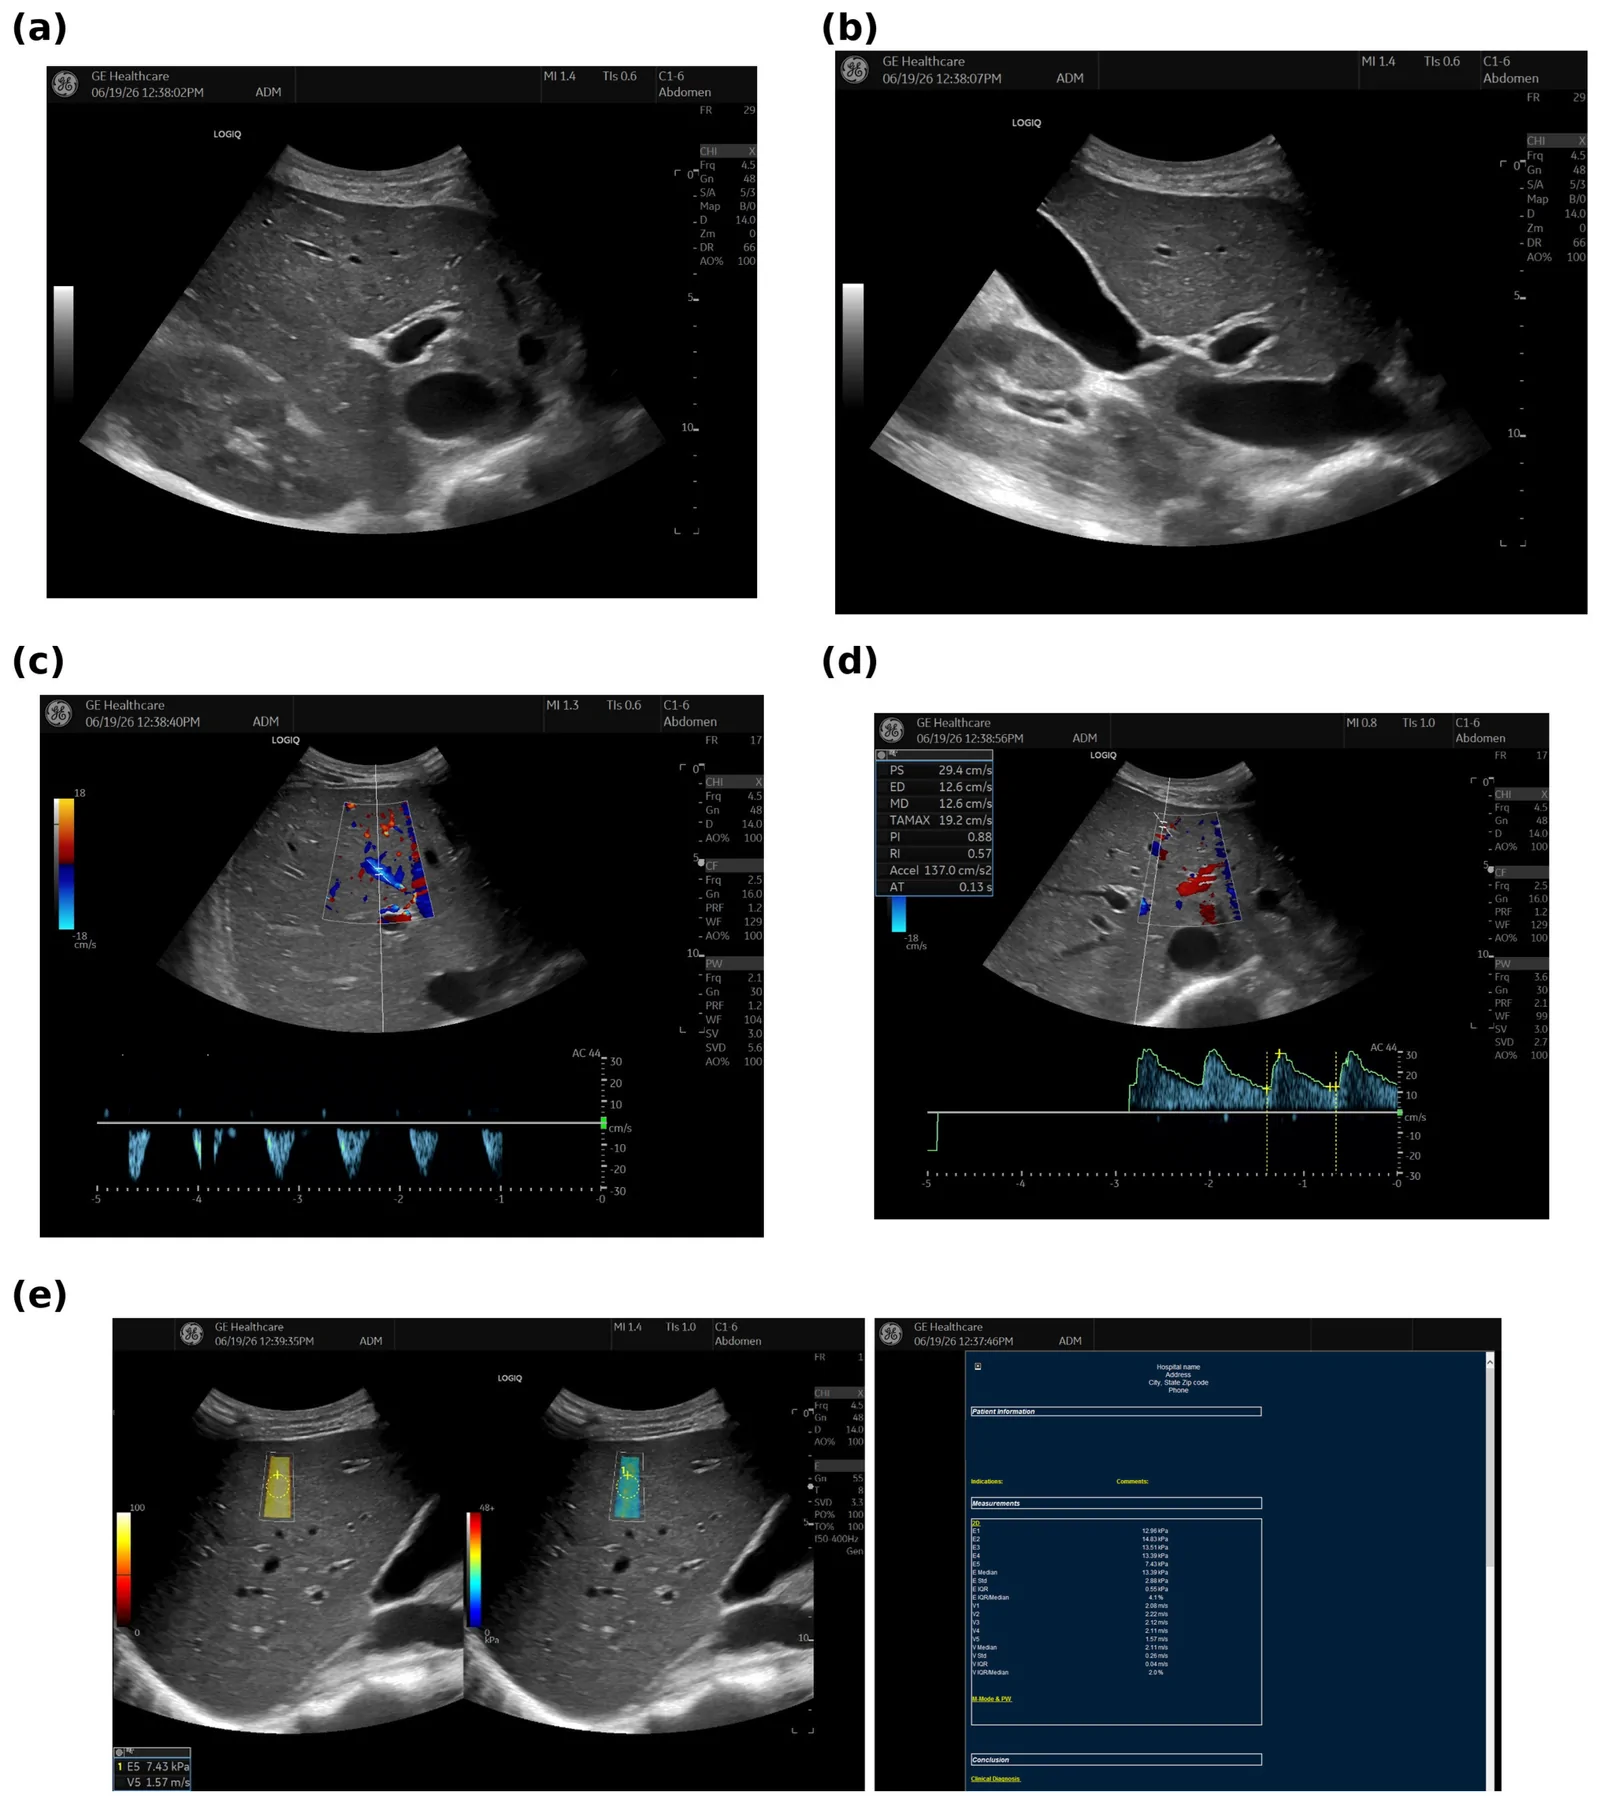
Ultrasound findings in chronic hepatitis C. (a) B-mode image showing mildly heterogeneous hepatic parenchymal echotexture. (b) Gallbladder wall thickening with periportal oedema. (c) Doppler assessment of the hepatic vein showing phasic flow variation related to the cardiac cycle. (d) Spectral Doppler of the hepatic artery showing normal antegrade flow and a normal resistive index (RI = 0.57). (e) Two-dimensional shear-wave elastography with the corresponding measurement detail, showing heterogeneous liver stiffness (median 13.4 kPa); stiffness heterogeneity should not be equated with a specific fibrosis distribution. In (c) and (d), the yellow dotted lines and cross marks are the machine-generated callipers from which the displayed spectral Doppler indices were derived, and negative values on the velocity scale denote flow directed away from the transducer. In (e), the colour overlays are the two-dimensional shear-wave elastography maps displayed on the system’s own scales, the yellow cross and dashed circle mark the measurement region of interest, and the adjacent panel lists the individual stiffness measurements obtained during the acquisition. All annotations and scales are generated by the ultrasound system and the images are reproduced without modification.
